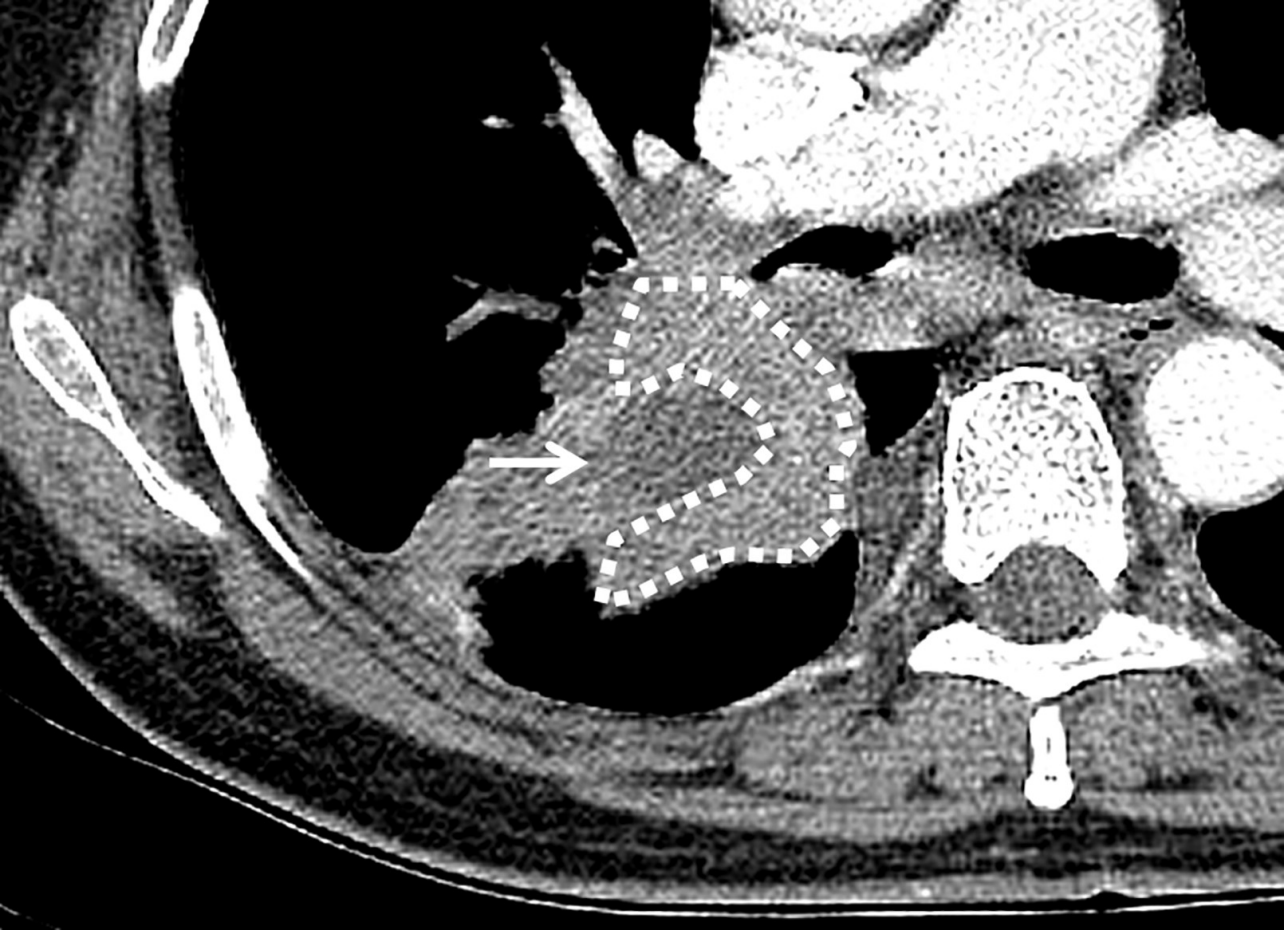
Placement of ROIs within the tumor. Regions of interest were drawn on the outline of the enhancing portion of the tumor (Polygon with a dashed margin). Areas showing necrosis (arrow) or cavitation were excluded from the ROI. ‘Heterogeneity’ was the standard deviation of CT values of the tumor, and ‘enhancement ratio’ was the mean CT value divided by that of the pectoralis muscle.
